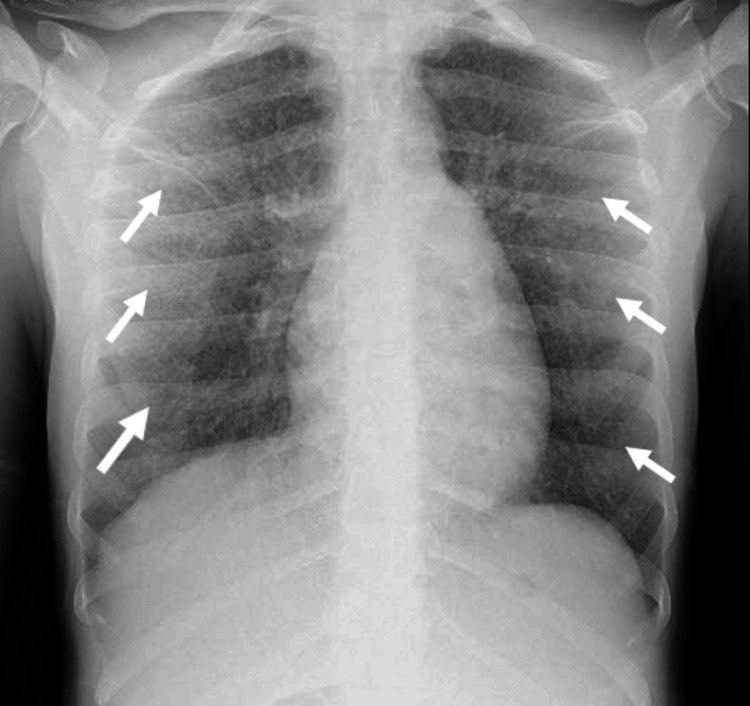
Chest X-ray showing miliary pattern.
